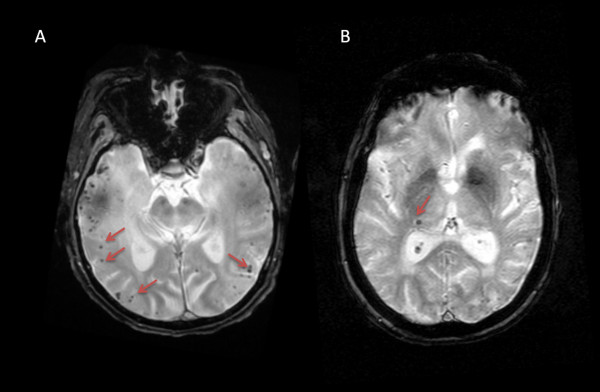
Cerebral microbleeds as seen on magnetic resonance imaging gradient-recalled echo imaging (arrows). (A) Multiple lobar microbleeds distributed across the temporal lobes. (B) Isolated deep microbleed in the lateral aspect of the right thalamus.
